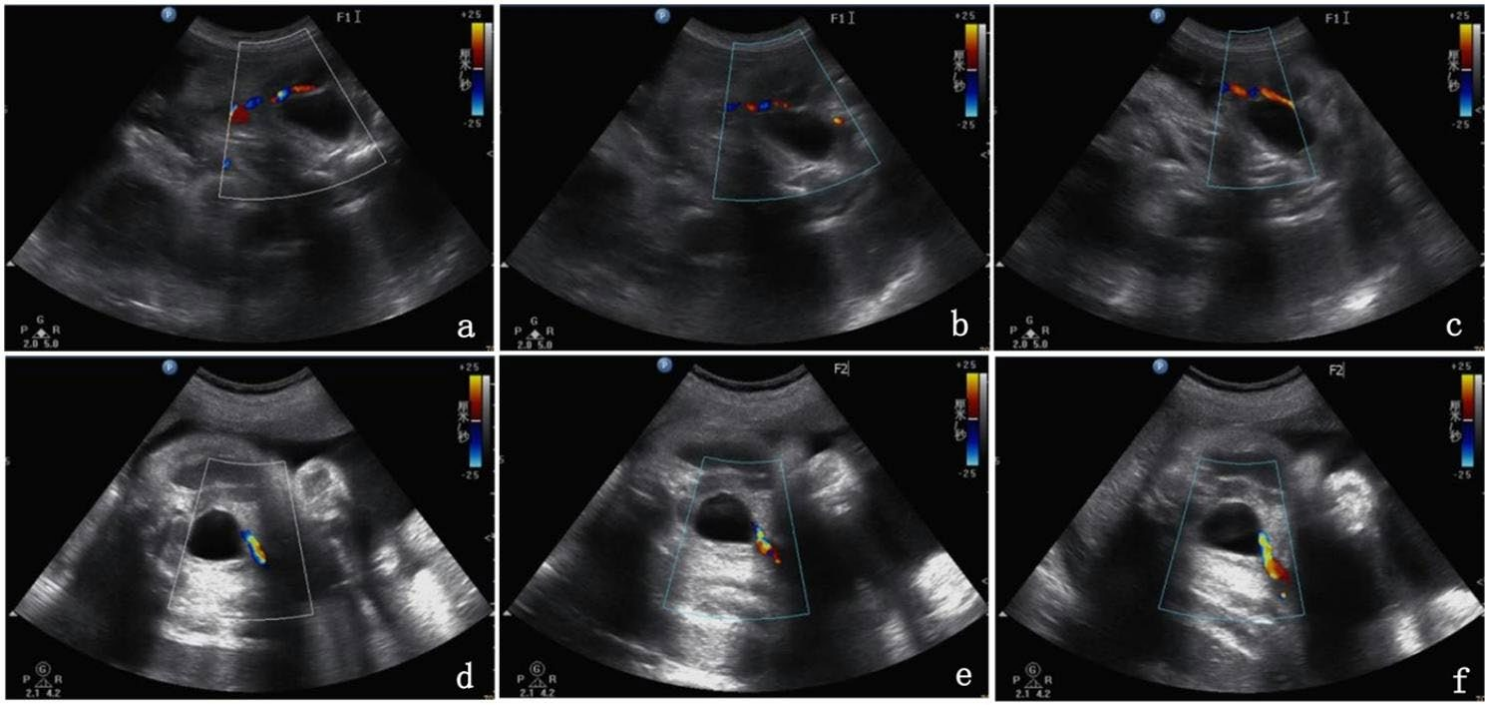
Cross-sectional views of ultrasound images at the level of the twins’ bladder at 31 + 6 weeks. The SUA blood flow images was shown on both sides of bladder of fetus A (a) and fetus B(d). Amidst continuous and dynamic umbilical artery blood flow observation and changing fetal position, there was still only one umbilical artery is visualized at the level of the bladder of fetus A (b,c) and fetus B (e,f). UAT should be seriously considered when only one umbilical artery is found for the first time in the third trimester
